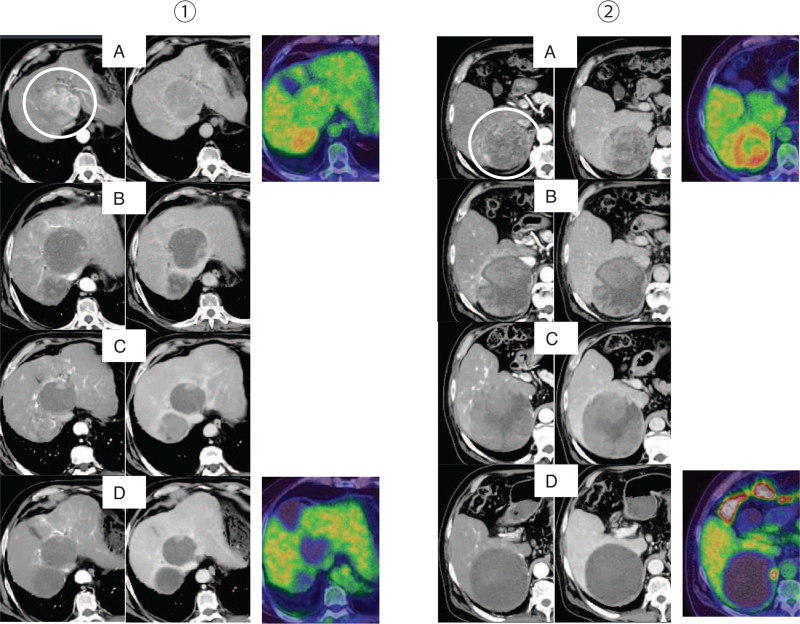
CT (early phase, delayed phase) and PET. (1) Intrahepatic HCC (S1) (2) Right adrenal metastasis. (A) CT and PET images at the initial diagnosis. (B) Six days after the first administration of atezolizumab plus bevacizumab. The internal contrast effects were diminished in both the intrahepatic HCC and the adrenal metastasis. (C) Evaluation of the efficacy after the fourth administration of atezolizumab plus bevacizumab. The intrahepatic HCC shrank while the adrenal metastasis grew larger than before, and the internal contrast effect was still present. (D) Evaluation of the efficacy of LEN after 1 month. The intrahepatic HCC maintained shrinkage, while the adrenal metastasis lost the internal contrast effect, and PET showed only light FDG uptake at the edge. CT = computed tomography, FDG = fluorodeoxyglucose, HCC = hepatocellular carcinoma, LEN = lenvatinib, PET = positron emission tomography.
